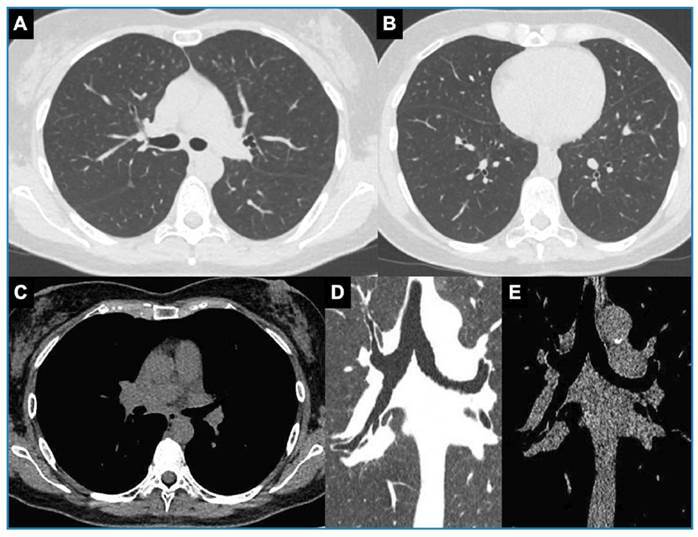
Chest computed tomography after completing fluconazole treatment. Axial images (A, B: lung window at two different levels; C: mediastinal window) and coronal images (D: lung window; E: mediastinal window) showing regression of the mass/lymph node conglomerate in the visceral compartment of the mediastinum at the subcarinal station, with a resolution of the endobronchial component and clearance of the parenchymal abnormalities in the right lung.
